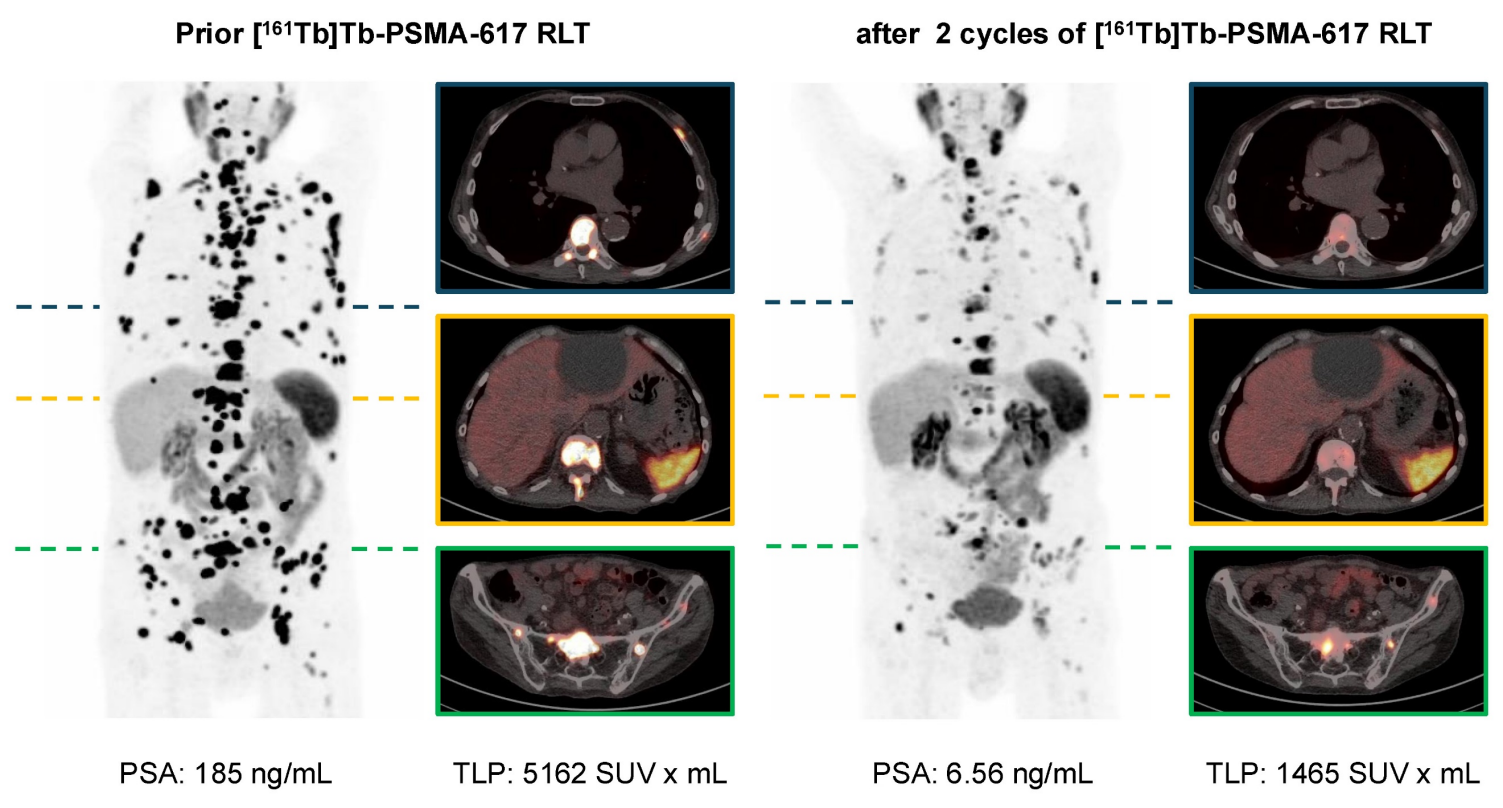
[^68^Ga]Ga-PSMA-11 PET/CT with maximum intensity projections (MIP) and transversal PET/CT slices of a patient with mCRPC undergoing [^161^Tb]Tb-PSMA-617 RLT. The patient experienced post-RLT progression after [^177^Lu]Lu-PSMA-617 RLT (initial 4 cycles); subsequently [^161^Tb]Tb-PSMA-617 RLT was initiated resulting in biochemical and molecular imaging partial remission (PR).
